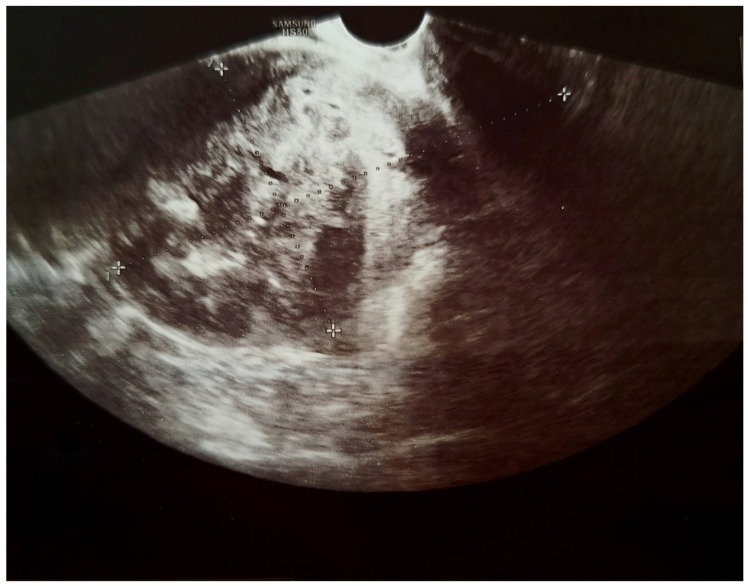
Transvaginal ultrasound image (longitudinal plane) showing an anteverted and anteflexed uterus measuring 106.3 × 65 mm.
